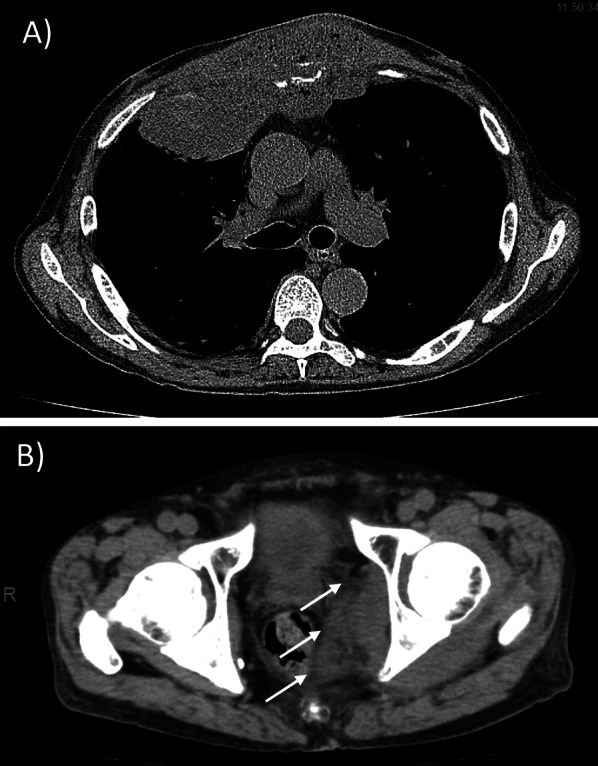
Computed tomography scans revealing multiple deep organ abscesses. A whole-body computed tomography (CT) scan showing an extensive abscess from the left subcutaneous into the right anterior mediastinum (A) and a sciatic rectus fossa abscess (B)
